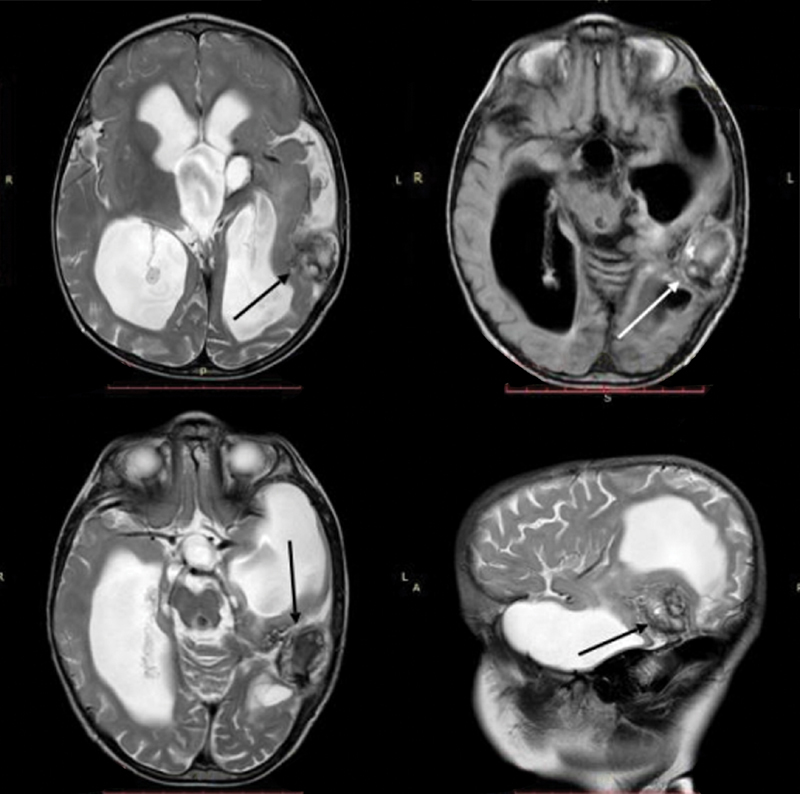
Brain magnetic resonance imaging axial T2-weighted images (
